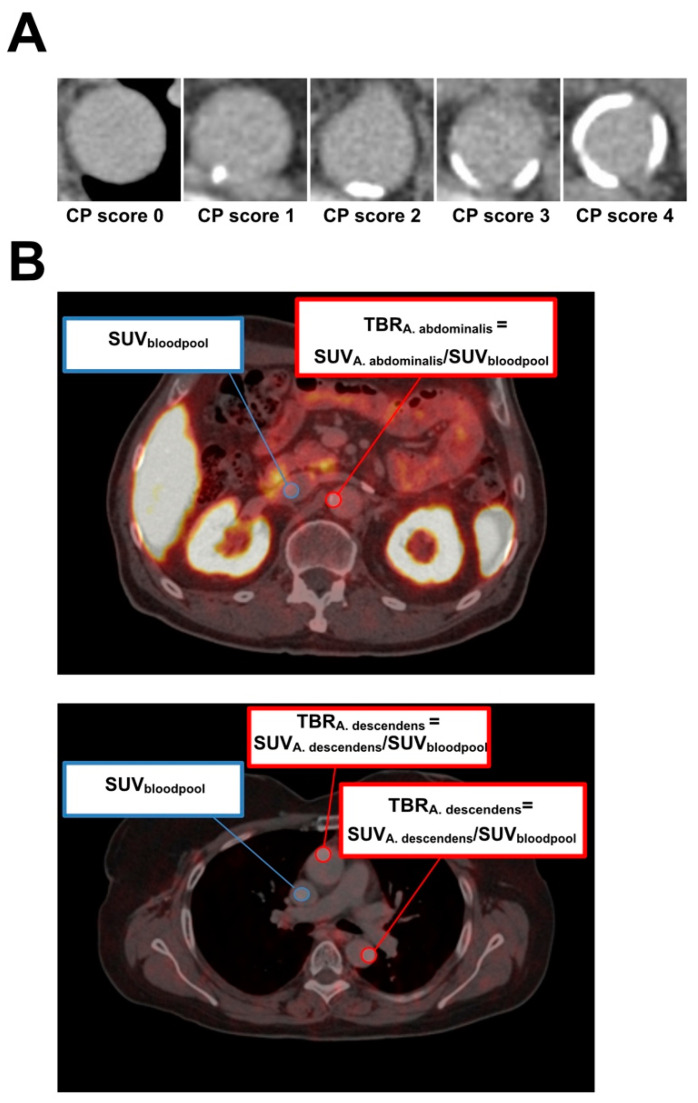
PET/CT image analysis. (A) Example of modified calcified plaque (CP) scoring system. Representative images show scores of 0 (no calcified plaque), 1 (calcified plaque involving 10% of vessel circumference), 2 (calcified plaque involving 10% to 25%), 3 (calcified plaque involving 25% to 50%), and 4 (calcified plaque involving >50%). (B) Representative image for target-to-background (TBR) calculation method. ROIs were drawn in the center of the vena cava inferior (blue) and arterial wall (red) for SUV evaluation.
